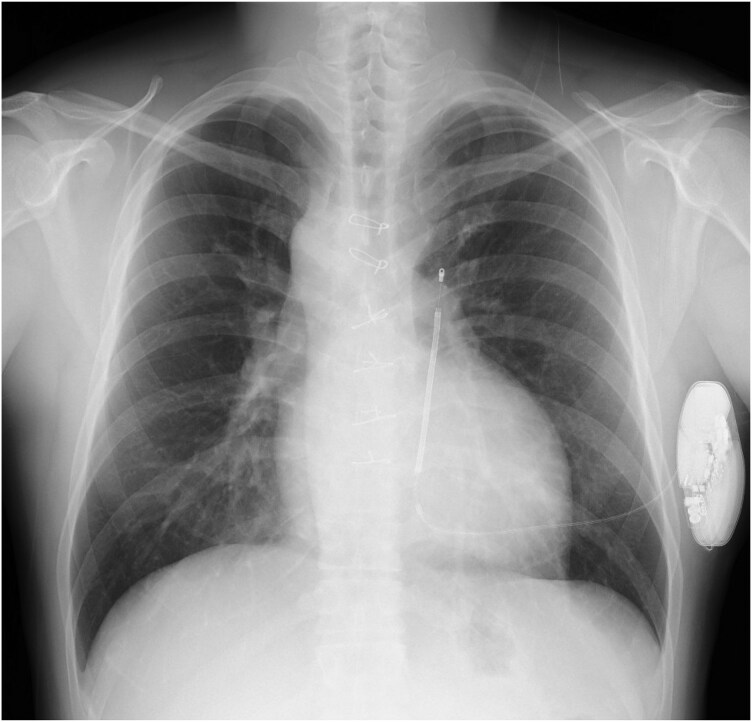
X-ray of the S-ICD. S-ICD, subcutaneous implantable cardioverter defibrillator.
